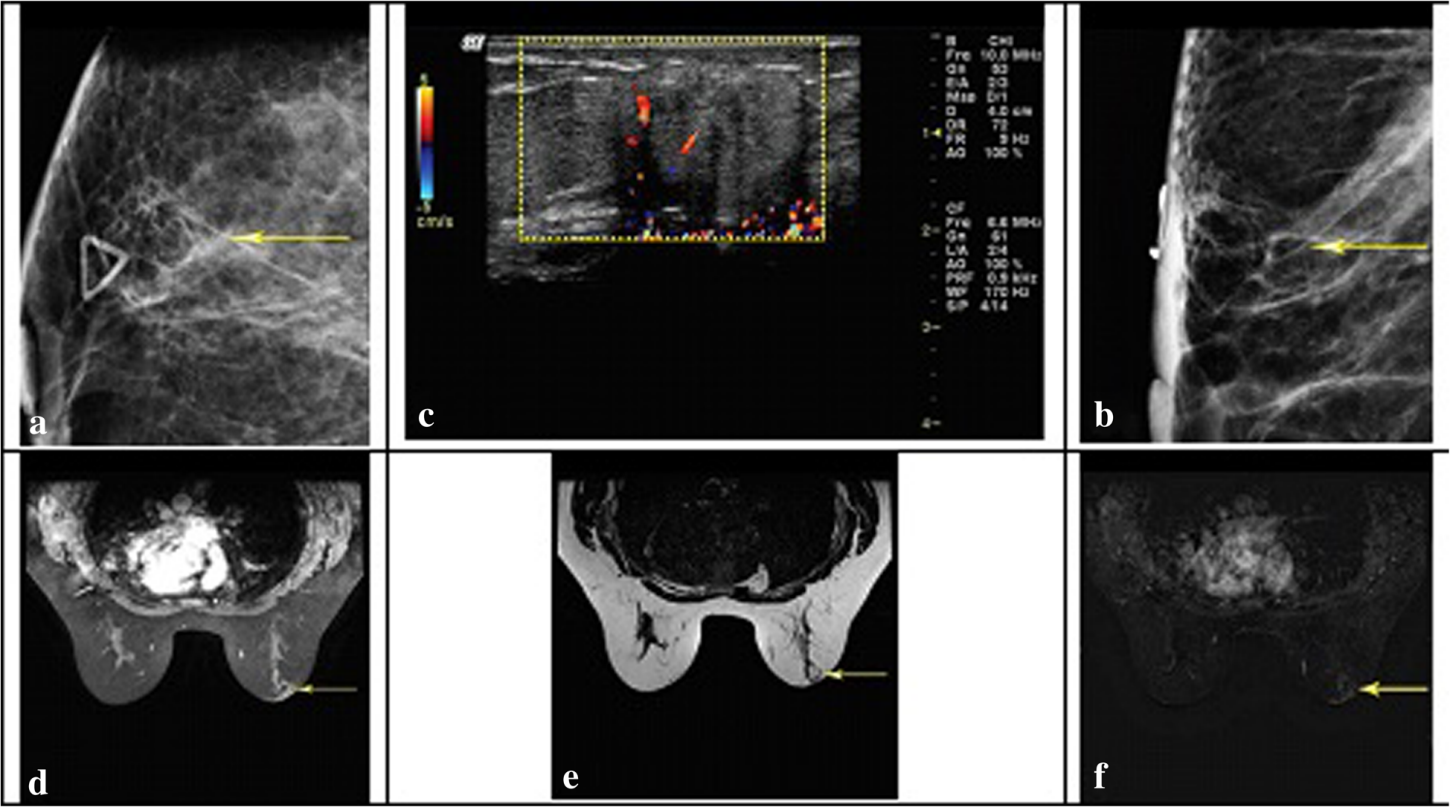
Craniocaudal projections and right breast mediolateral oblique. a and b) show a radiolucent lobular mass at site of palpable mass (arrow). c) Targeted ultrasound at site of palpable mass demonstrates a lobular heterogeneous hypoechoic mass with posterior acoustic shadowing. d) Axial T1-weighted fat saturation after gadolinium. e) T2-weighted nonfat saturation, and f) subtraction images that indicate a mass at 11 o’clock in the right breast anteriorly that follows fat signal on all sequences with thin rim enhancement (Derived in accordance with William et al. [19])
